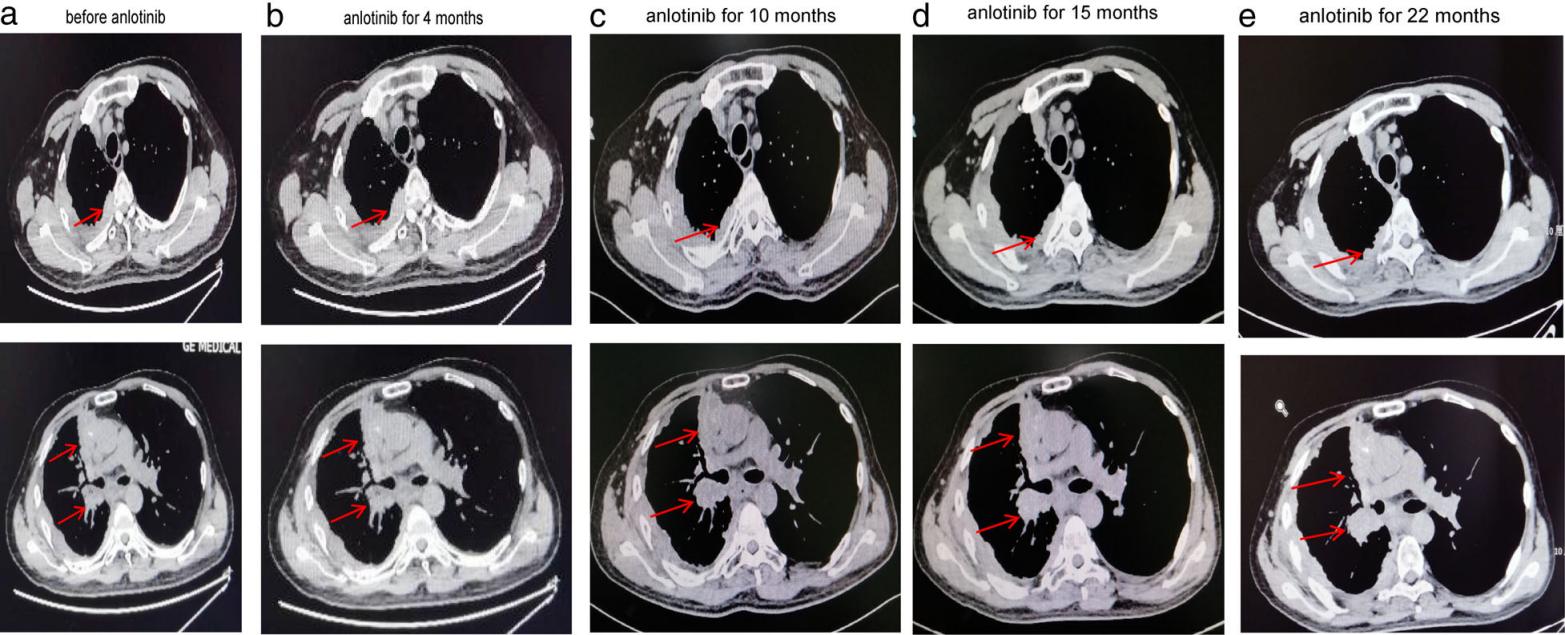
Chest computed tomography (CT) scans before and after anlotinib therapy. (a) Chest CT of different layers taken before anlotinib therapy revealed a soft tissue mass in the mediastinum and multiple metastases in the lymph nodes of the right hilum and mediastinal, right pleura, and right lung. (b) After four months of anlonitib treatment, chest CT showed that the mediastinal mass and metastatic lesions remain basically stable compared to the baseline. (c) After 10 months of anlotinib treatment, there was little change in the mediastinal mass and metastatic lesions on CT compared with the previous scan. (d) After 15 months of anlotinib treatment, the mediastinal mass and metastatic lesions remain stable. (e) To date, 22 months of PFS have been achieved.
